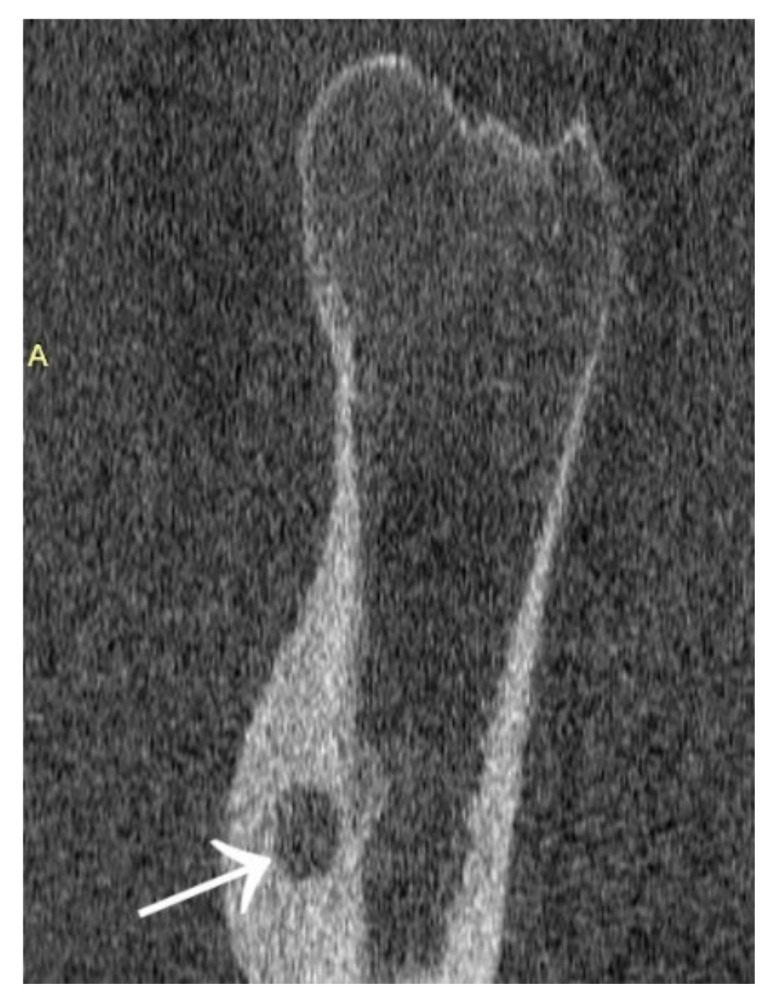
CT scan showing a focus of OO (arrow) in the left lesser trochanter.
